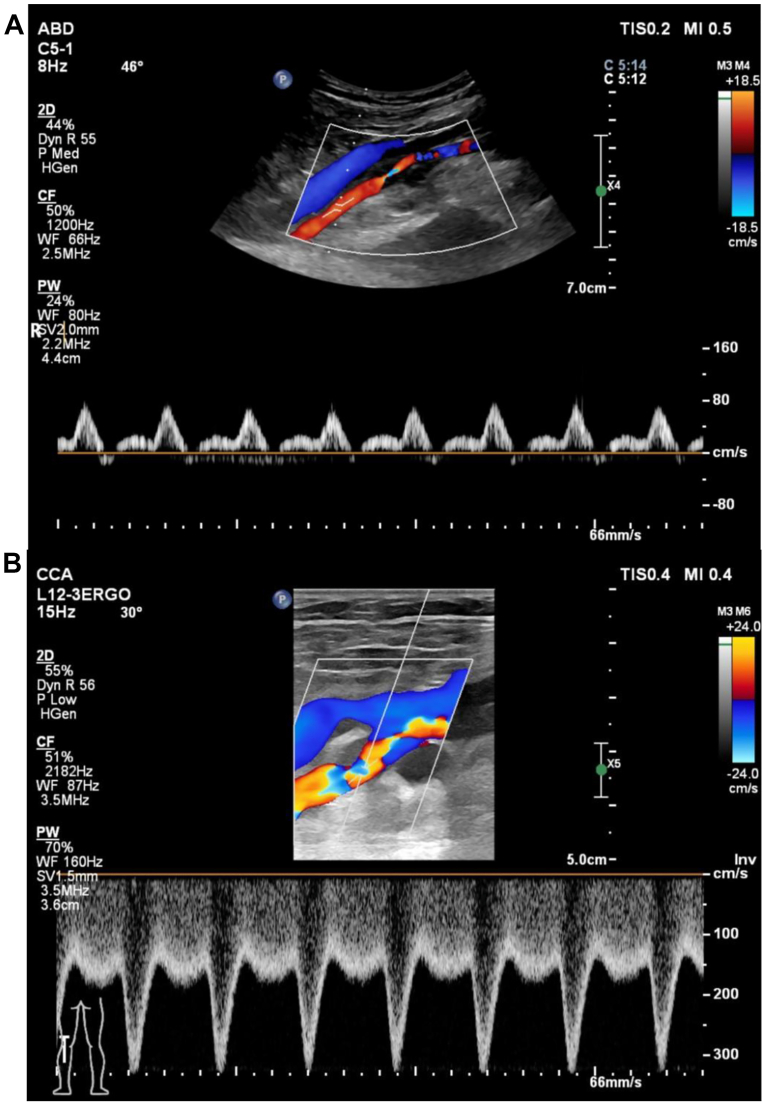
(A) The Doppler ultrasound scan showed the peak systolic velocity (PSV) of the proximal segment of the popliteal artery at the site of compression was less than 80 cm/s. (B) The Doppler ultrasound scan indicated that the blood flow velocity at the site where the popliteal artery was compressed increased, with the PSV exceeding 300 cm/s, although the standard criteria define a PSV over 275-300 cm/s as indicative of severe stenosis, typically 70%-99% narrowing.
